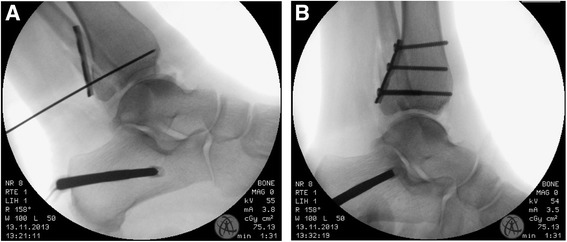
Intraoperative x-ray; a Lateral view with temporary k-wire fixation of the posterior fragment; b Lateral view after fixation with a three-hole one-third tubular plate for fixation of a posterior malleolus fracture
